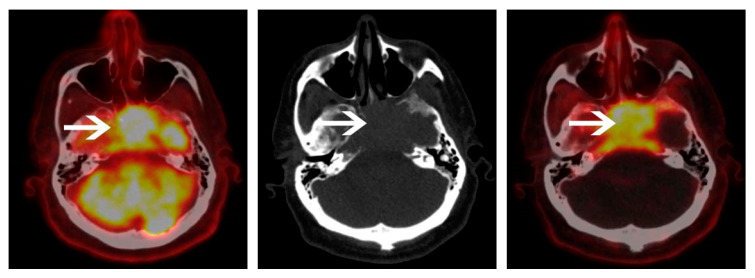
A 65-year-old male with a light chain multiple myeloma (MM). ^18^F-fluorodeoxyglucose glucose (^18^FDG) positron emission tomography/computed tomography (PET/CT) (left panel) and ^18^F-Choline PET/CT (right panel) were performed with a 4-day interval. ^18^F-Choline PET/CT axial image of the skull shows an intense uptake of a skull base lesion. Because of the intense surrounding cerebral uptake on ^18^FDG PET/CT, the lesion is more difficult to individualize. Clinical examination at baseline identified the presence of diplopia and left ptosis, which disappeared a few days after induction chemotherapy was started.
